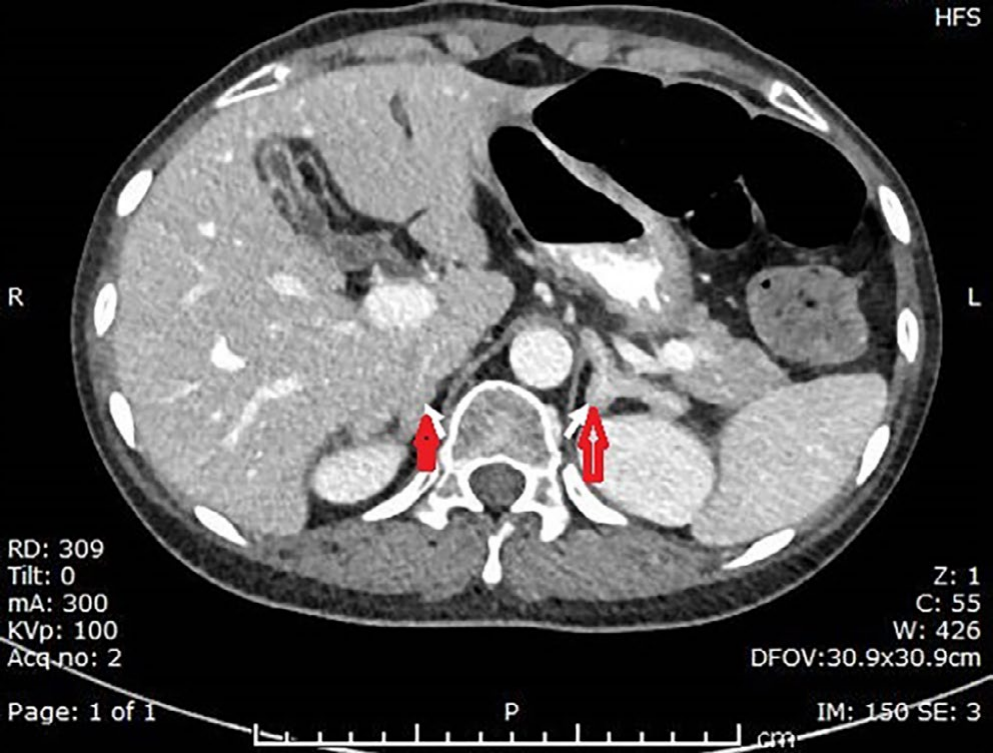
Axial contrast‐enhanced CT study at the level of the upper abdomen showing a smooth enlargement of both adrenal glands in keeping with hypertrophy (arrows)
